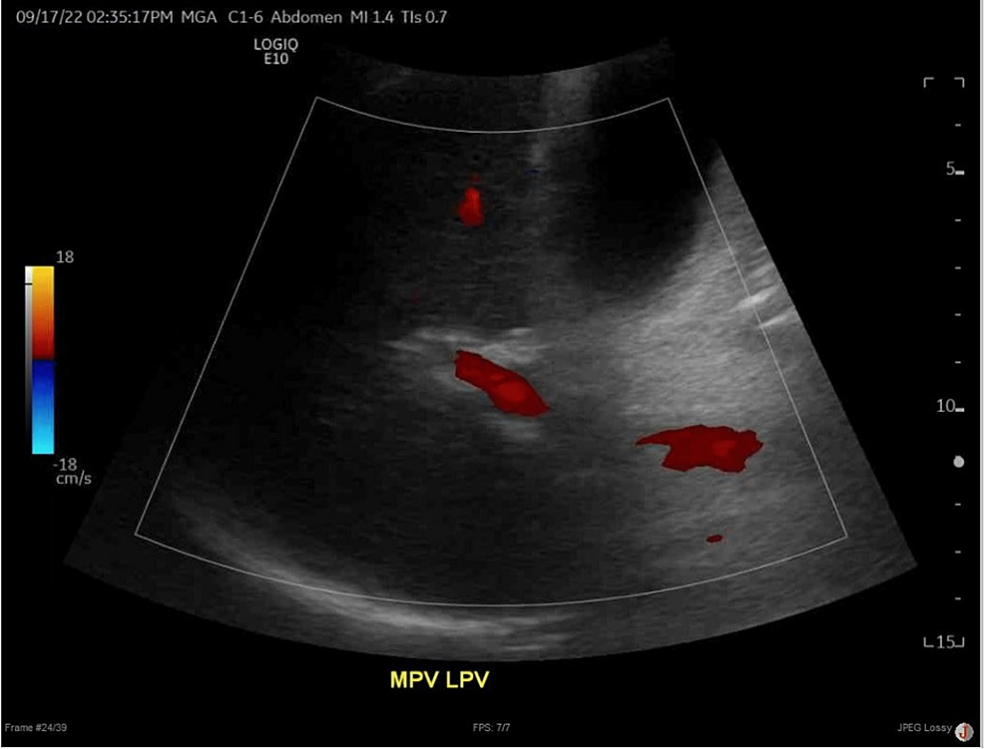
Duplex ultrasonography demonstrating patent hepatic and portal vasculature with appropriate directional flow and duplex waveforms.
